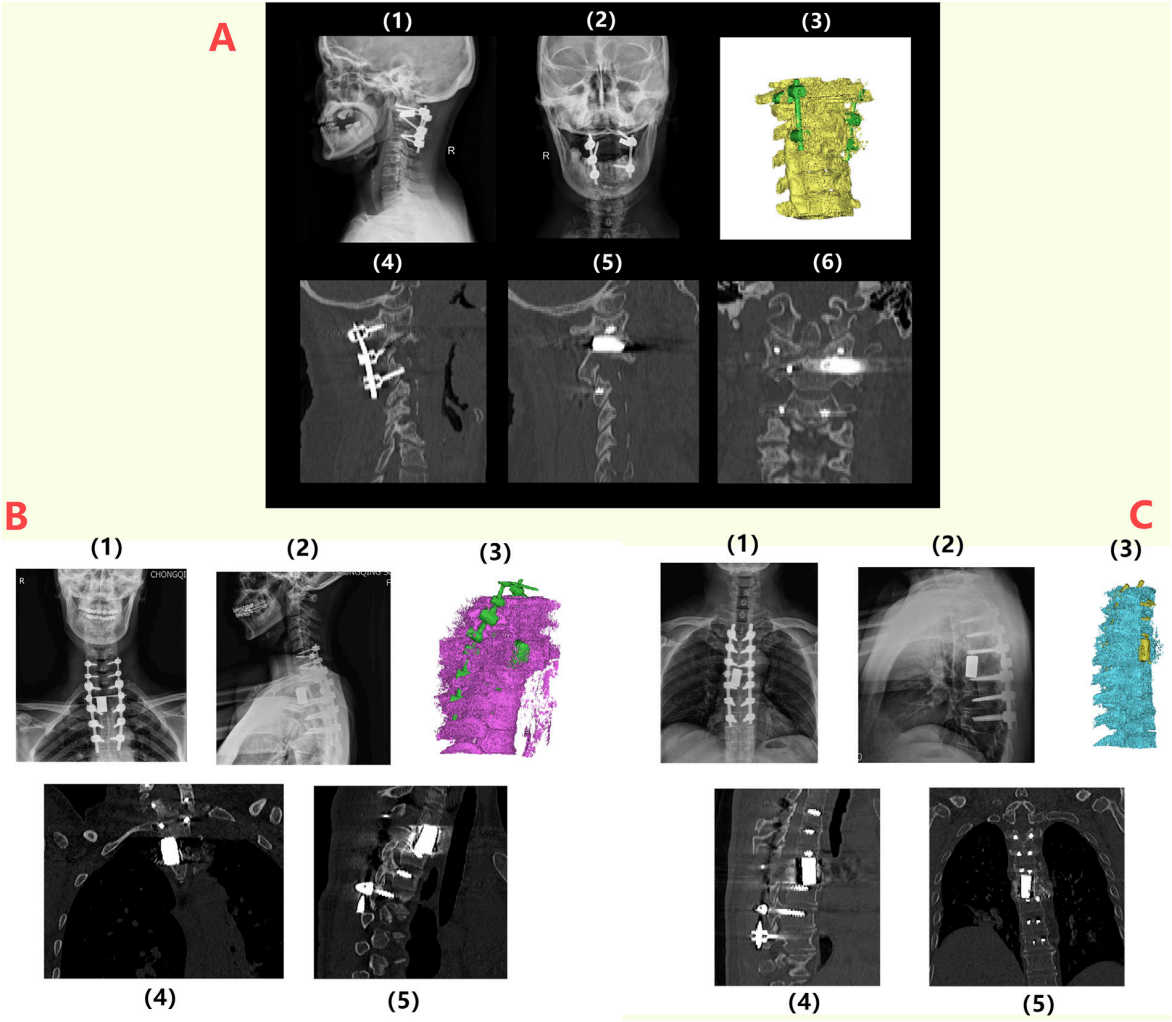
Postoperative radiographic follow-up of patients: (A) 1/2: Lateral and anteroposterior X-ray images of the spine of Patient 1 at 6 months postoperatively, indicating the proper positioning of the prosthesis and internal fixation; 3/4/5/6: CT images and three-dimensional reconstruction at 6 months postoperatively, showing no loosening or displacement of the prosthesis and internal fixation; (B) 1/2: Lateral and anteroposterior X-ray images of the spine for Patient 3 at 6 months postoperatively, indicating the proper positioning of the prosthesis and internal fixation; 3/4/5: CT images and three-dimensional reconstructions at 6 months postoperatively, showing no loosening or displacement of the prosthesis and internal fixation; (C) 1/2: Lateral and anteroposterior X-ray images of the spine for Patient 2 at 6 months postoperatively, indicating the proper positioning of the prosthesis and internal fixation; 3/4/5: CT images and three-dimensional reconstructions at 6 months postoperatively, showing no loosening or displacement of the prosthesis and internal fixation.
